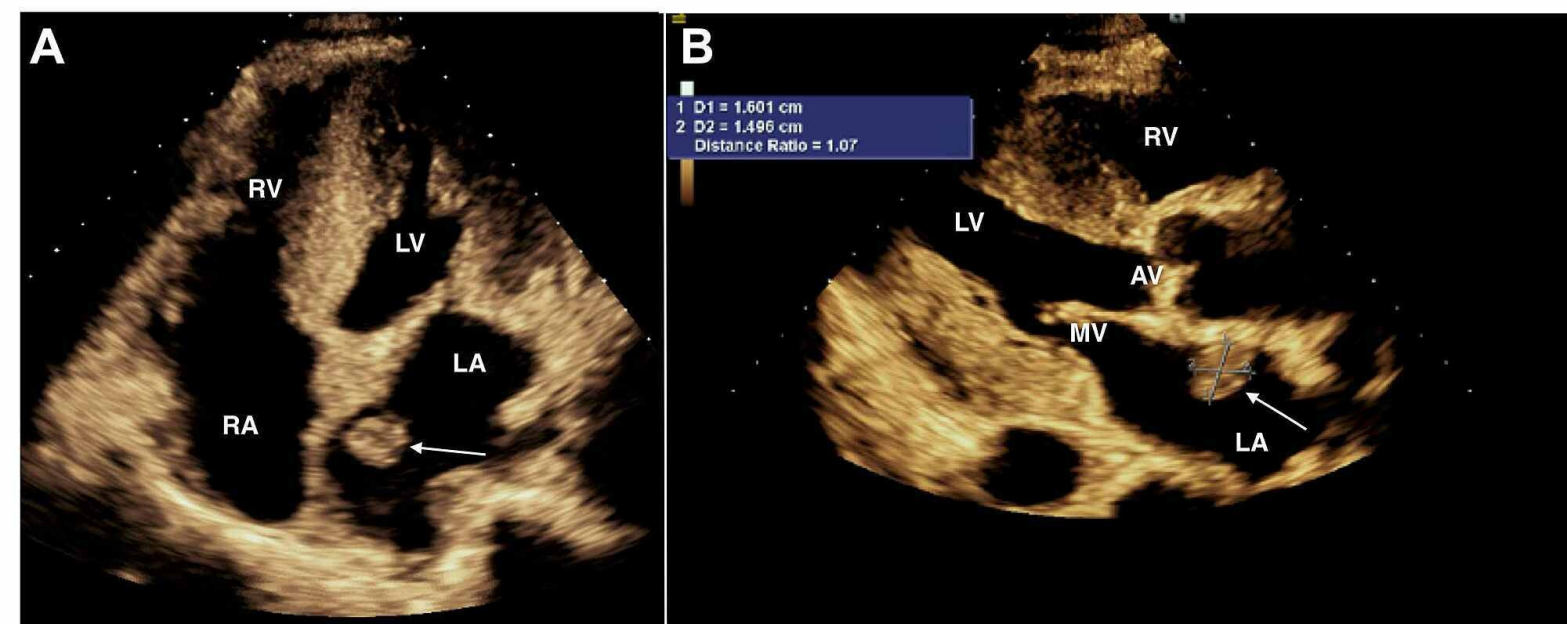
Transthoracic echocardiogram parasternal long axis and apical four-chamber view (A) Transthoracic echocardiogram apical four-chamber view revealing left atrial echocardiogram density denoted with a white arrow; (B) transthoracic echocardiogram parasternal long-axis view revealing 1.49 × 1.61 cm^2^ left atrial pedunculated echodensity, attached to the posterior wall of the left atrium denoted with the white arrow. LA: left atrium, LV: left ventricle, RA: right atrium, RV: right ventricle, MV: mitral valve, AV: aortic valve.
